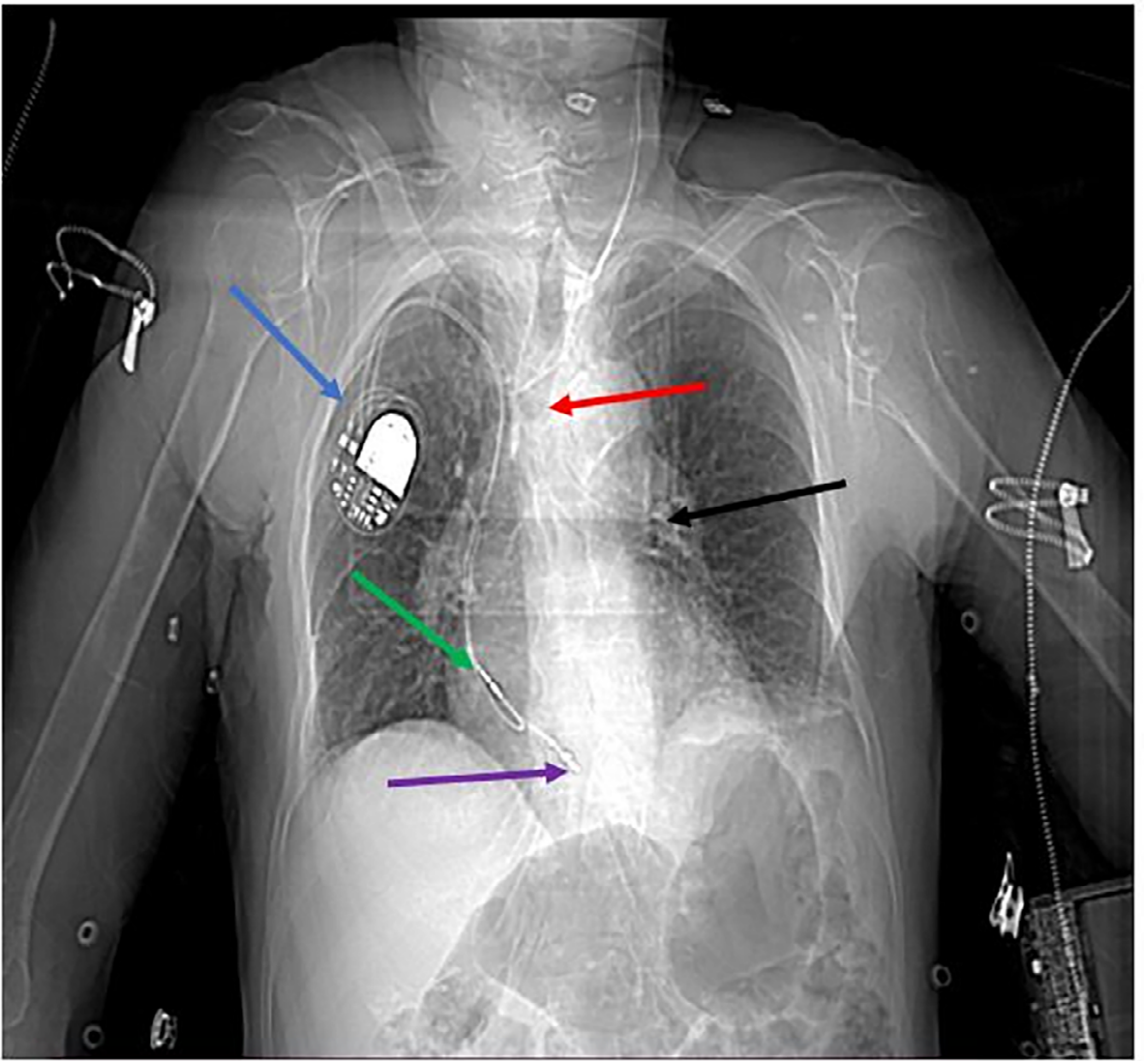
Chest X-ray showing pacemaker position (blue arrow), lead Pathway (green/purple arrows), tracheal Deviation (red arrow), and Foreign Body (black arrow).
